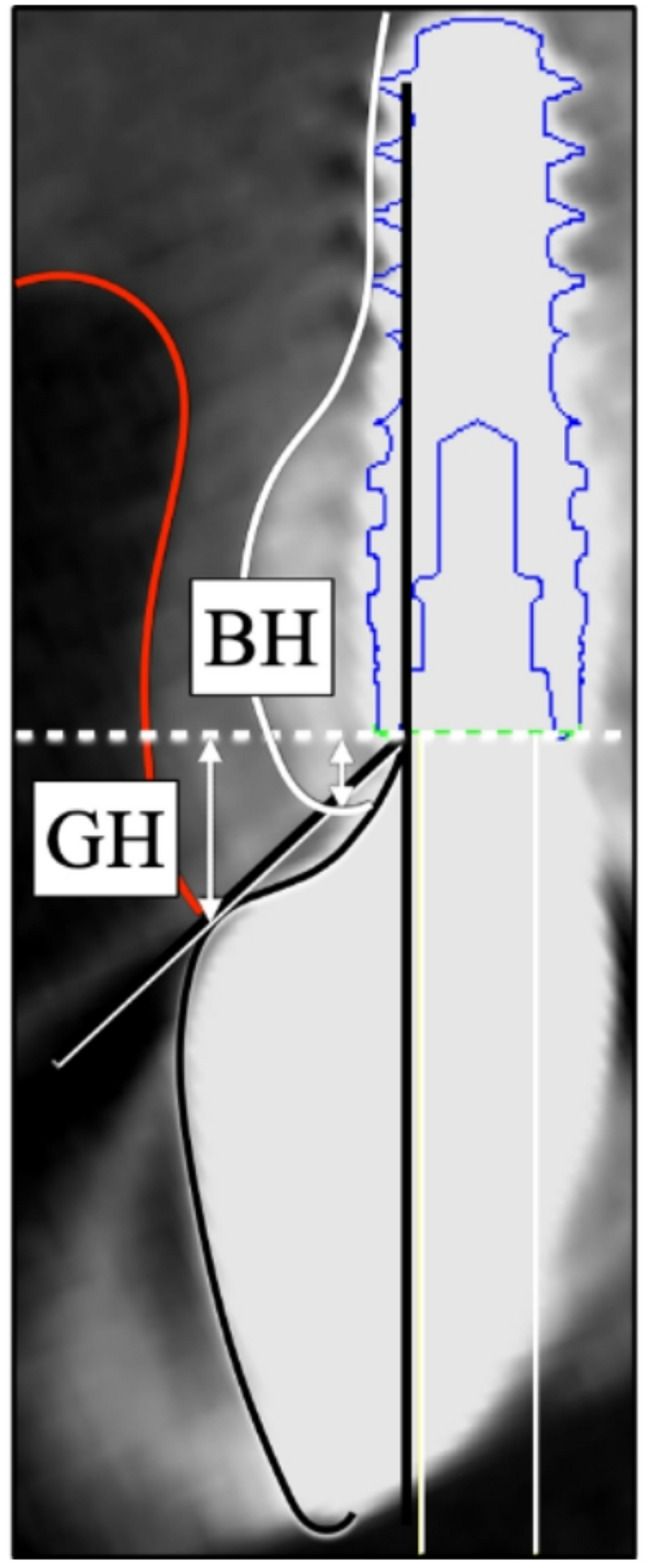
Measurements of labial transmucosal tissue morphology on CBCT images at superstructure placement and 1 year after placement. Abbreviations: GH, gingival height from platform; BH, bone height from platform; EA, emergence angle; SCD, subgingival contour distance
